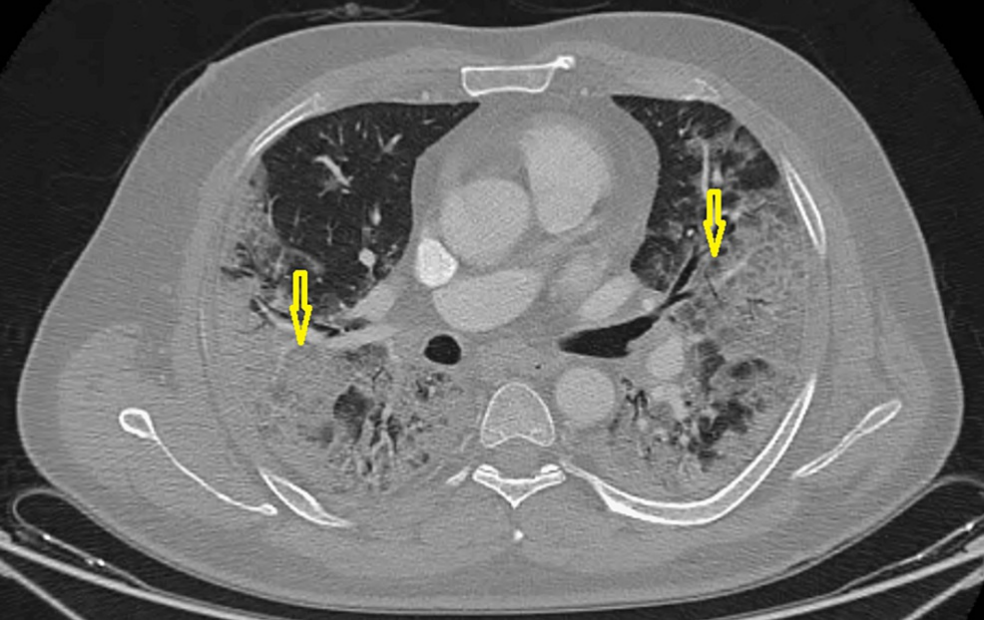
Preoperative chest computed tomography on transverse thin-section scans showing extensive ground-glass opacities of both lungs (yellow arrows)
